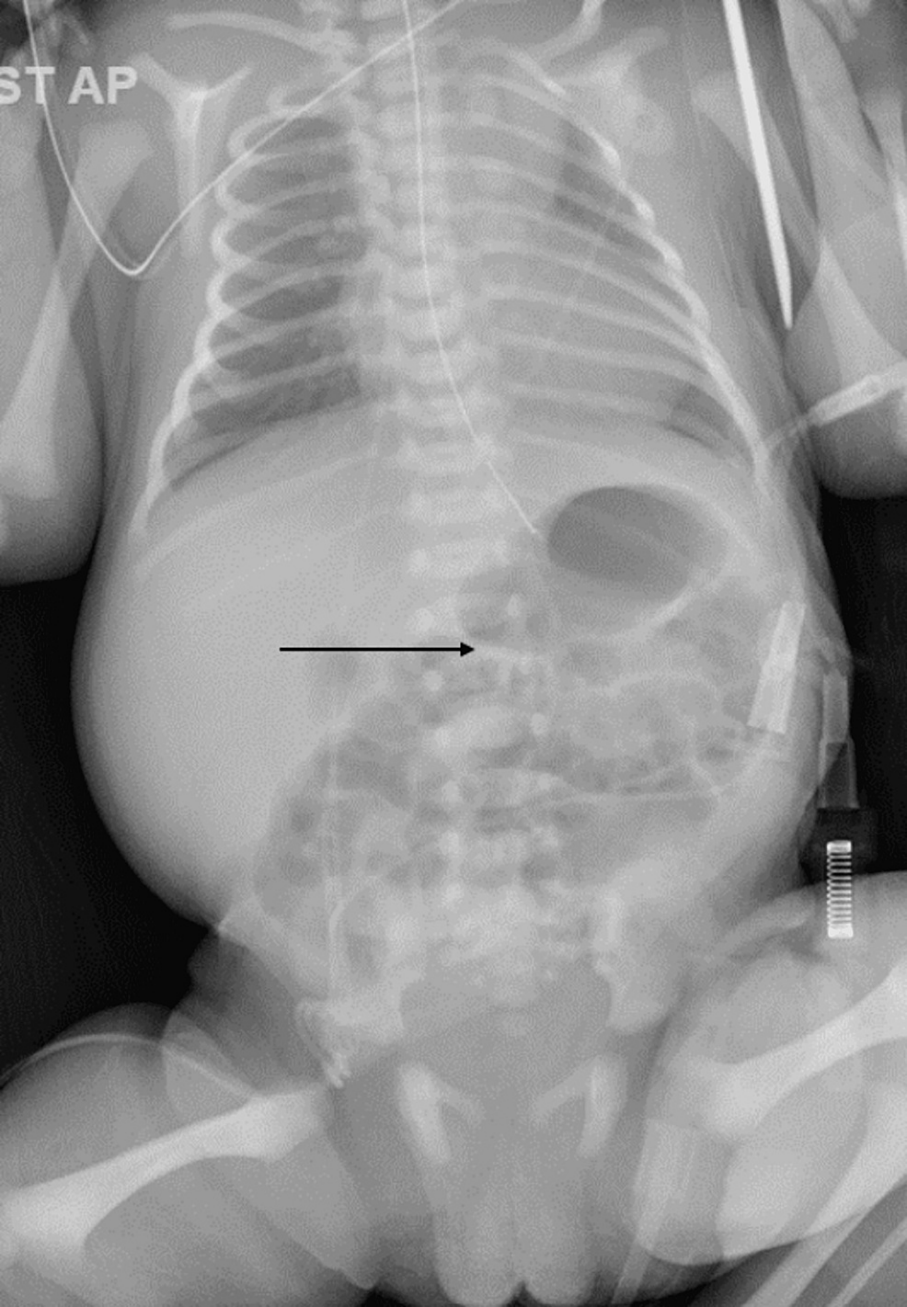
Abdominal X-ray with normal bowel gas pattern and no dilated bowel loops visualized (arrow)
